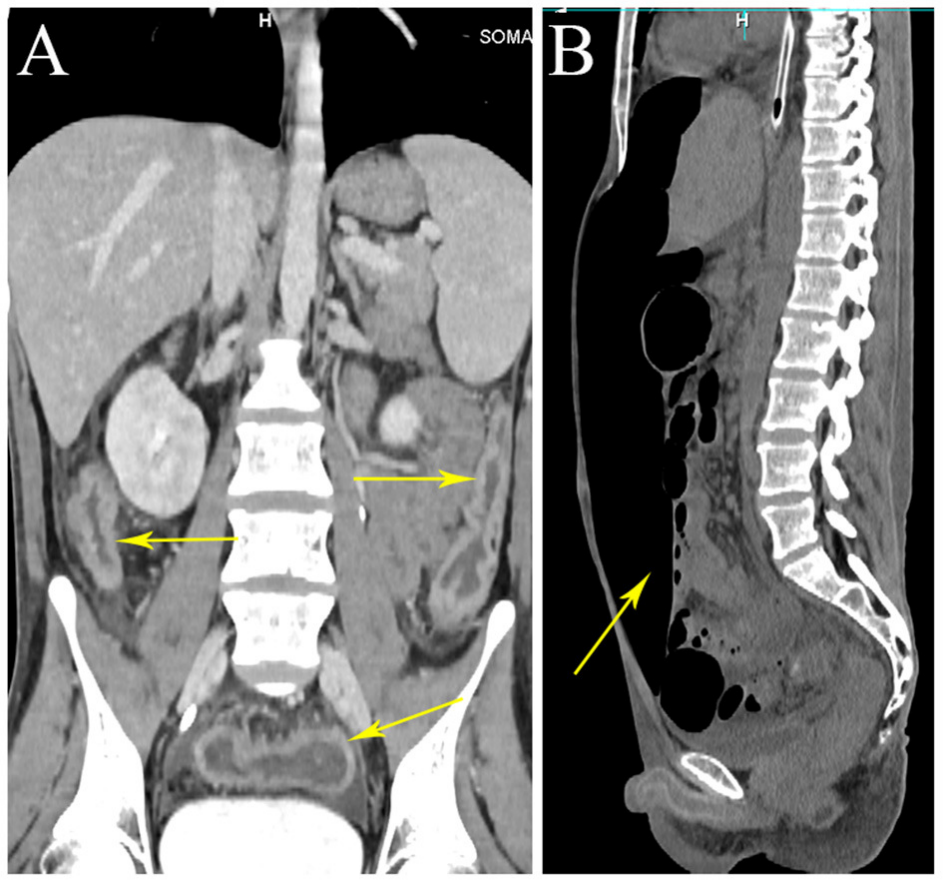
Whole abdominal CT. CT scan showing wall thickening of the colon (arrows). CT scan showing a large amount of free gas in abdominal cavity (arrow). (A) Coronal view. (B) Sagittal view.
